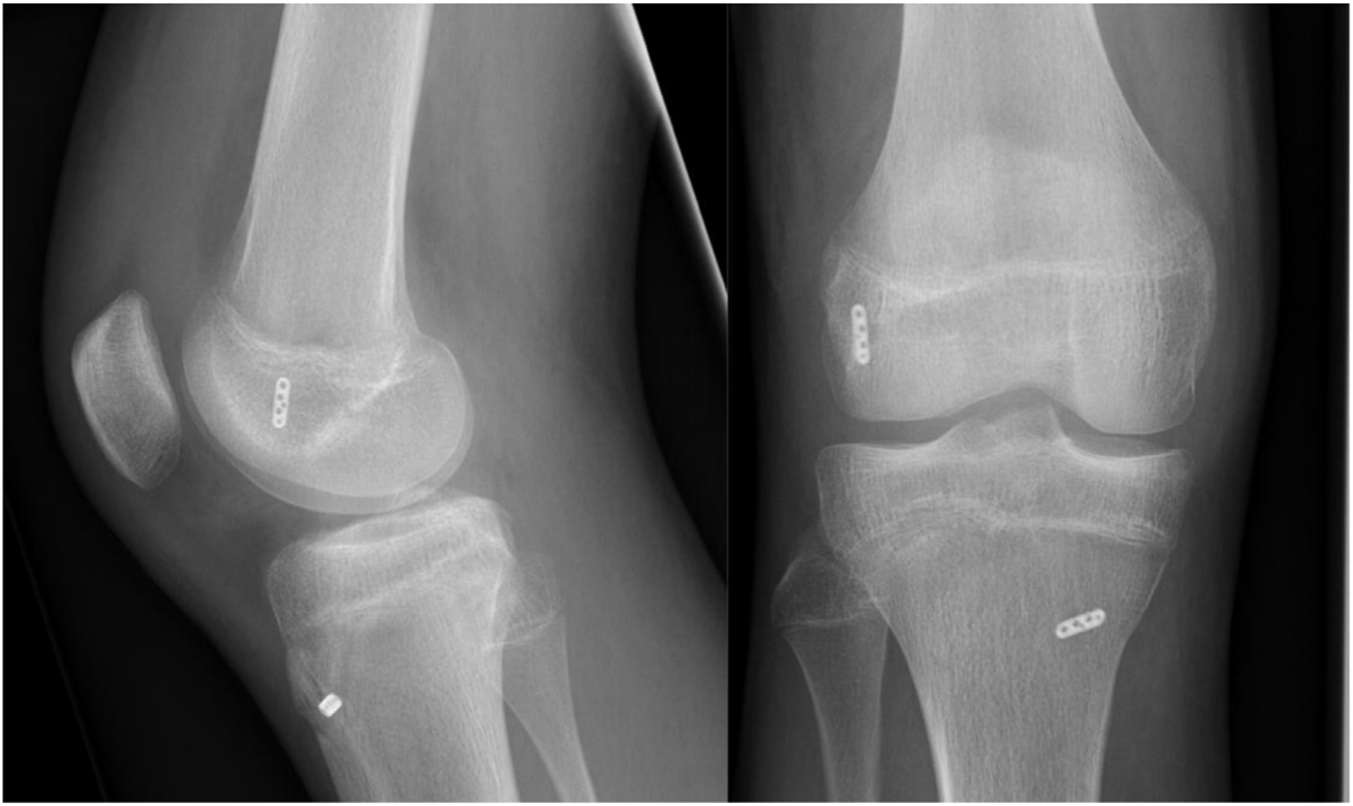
All operated patients underwent postoperative radiography in sagittal and frontal plane to confirm the correct position of the tunnels.
